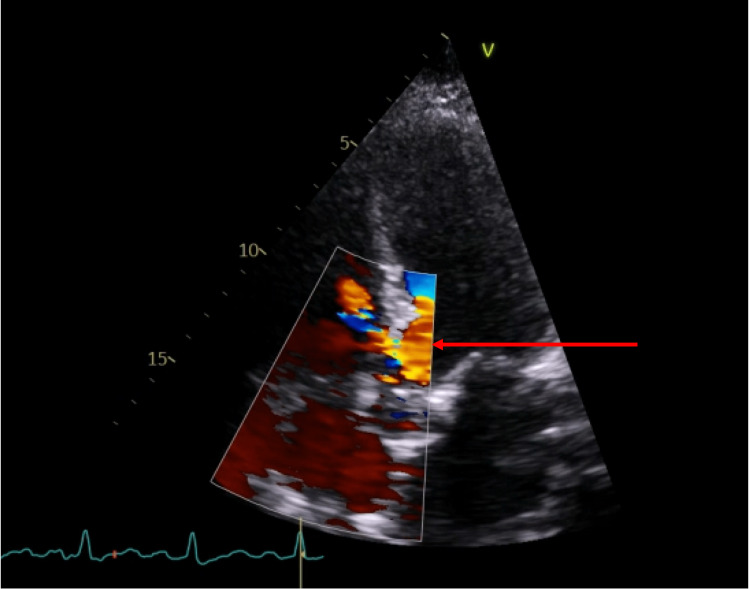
Apical five-chamber view of a transthoracic echocardiogram with colour Doppler indicating flow across the acquired ventricular septal defect.
